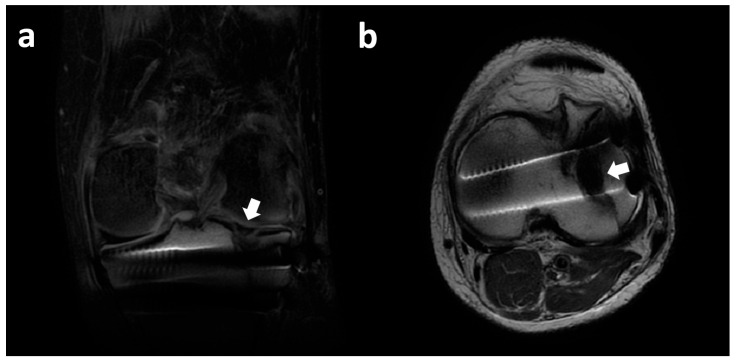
Left knee coronal fat-suppressed PD-weighted MR image (a) and axial T2-weighted MR image (b) show a portion of the lateral meniscus entrapped in the fracture rim of the tibial plateau joint face (white arrows).
